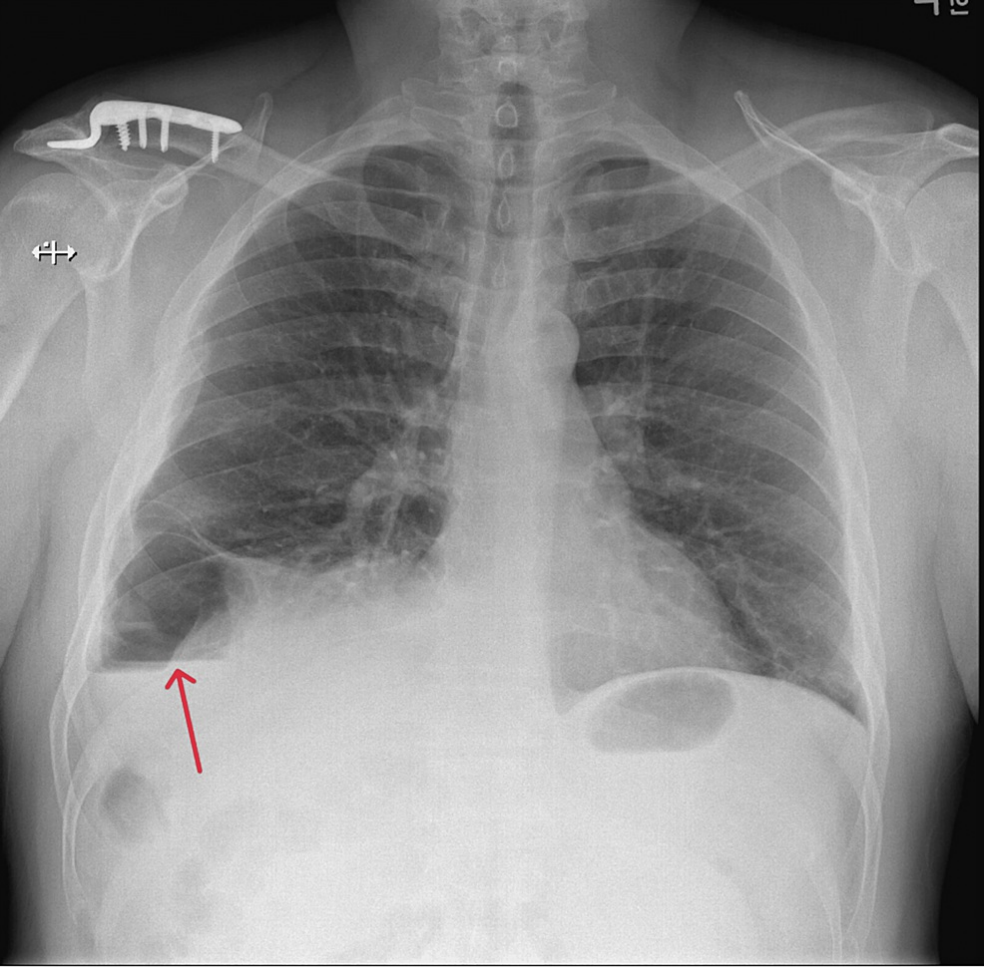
Chest x-ray showing ill-defined right lung base. Red Arrow: air-fluid level
